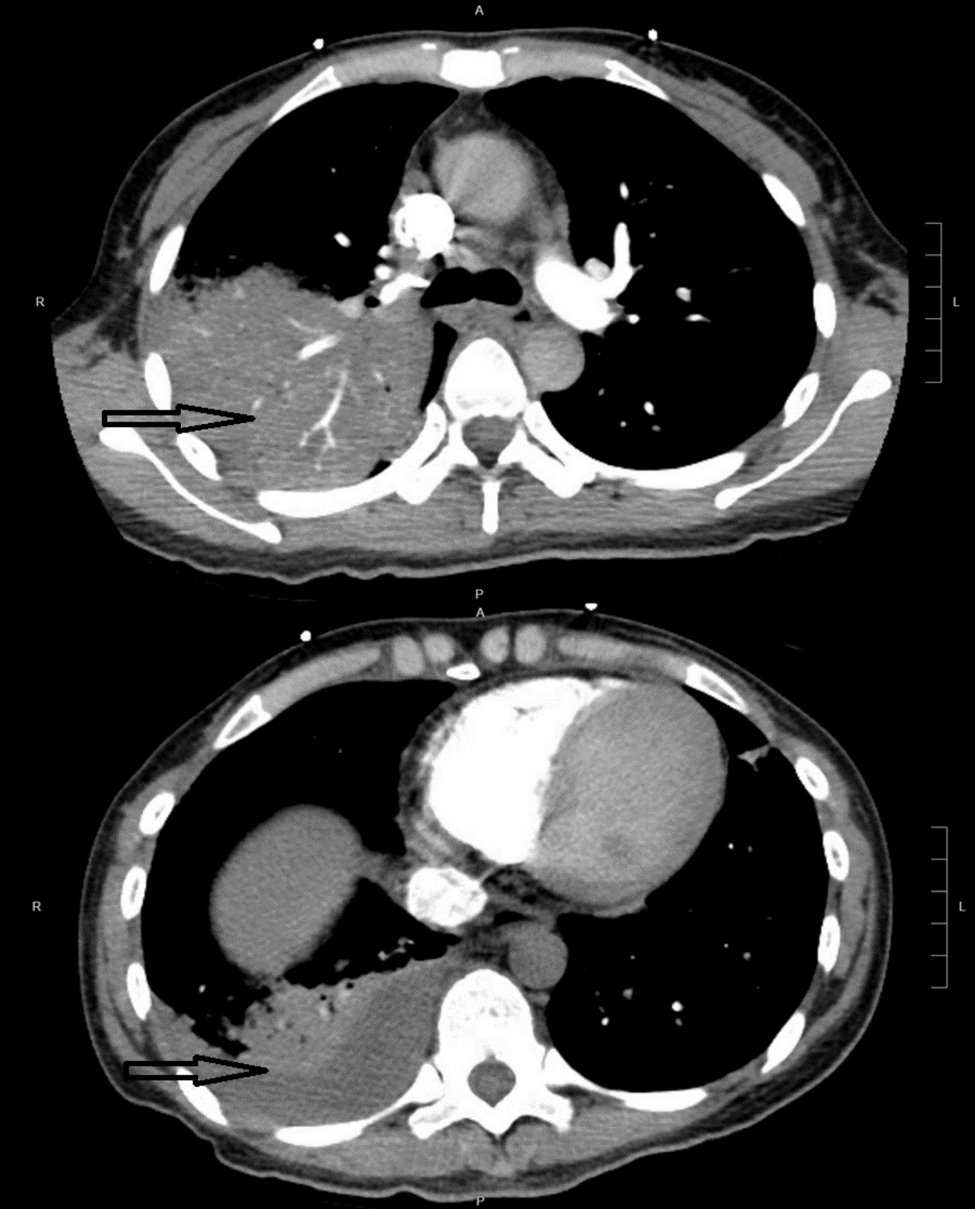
Computed tomography pulmonary angiogram images of Case 1 showing right upper lobe consolidation and a small right pleural effusion.
